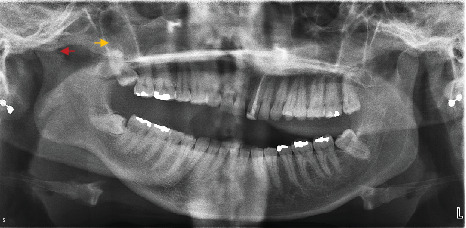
Orthopantomography of the patient. Right coronoid process hyperplastic (yellow arrow). Right condyle hypoplastic (red arrow).
